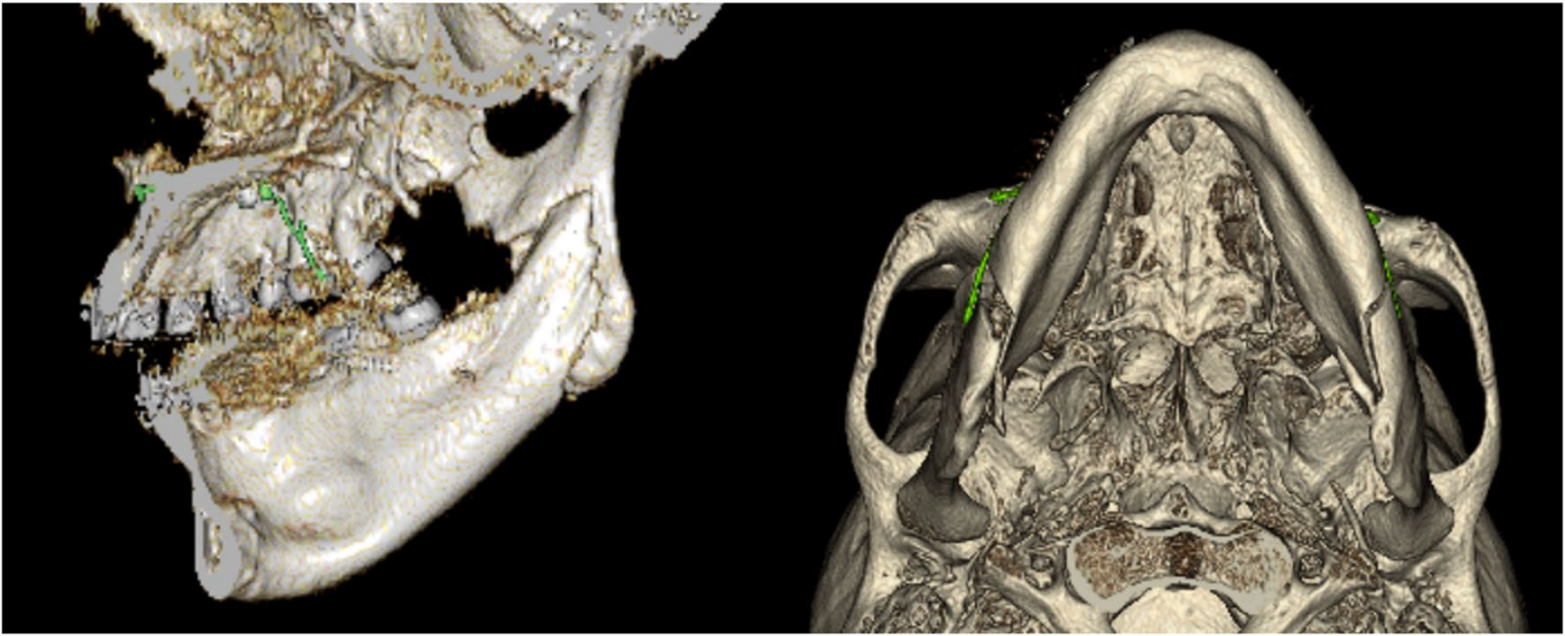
CT images showing SS. A vertical fracture line extending from the lingula to the inferior border of the mandible can be seen
